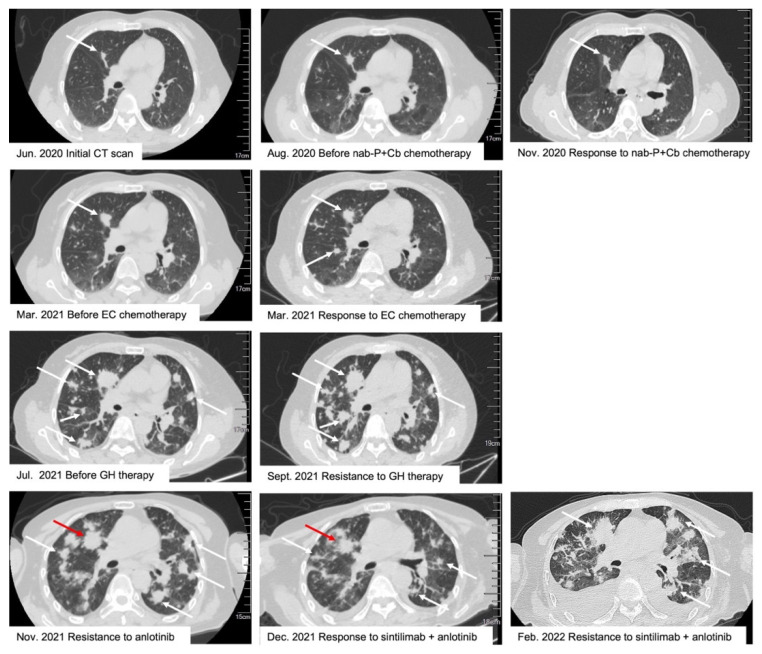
Computed tomography scan images of the patient’s clinical course. Abbreviation: nab-P: nab-paclitaxel; Cb: carboplatin; EC: epirubicin/cyclophosphamide; GH: gemcitabine/trastuzumab. (The red arrow shows the location of the target lesion. The white arrow shows the location of the non-target lesion).
